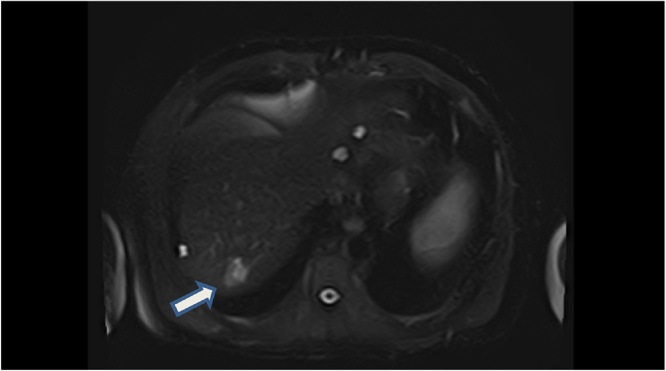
Axial T2 Fat-suppressed MRI image of liver. White arrow showing posterior segment VII lesion.
